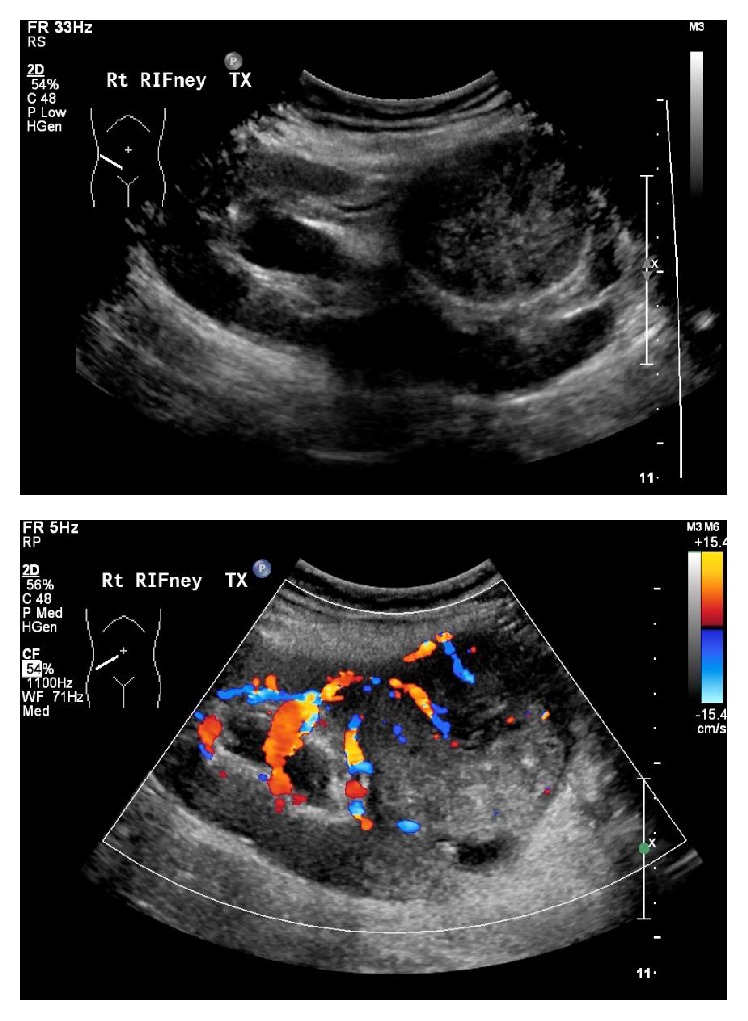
Ultrasound imaging showing a solid mass arising from the midpole of the renal allograft with internal vascularity.
